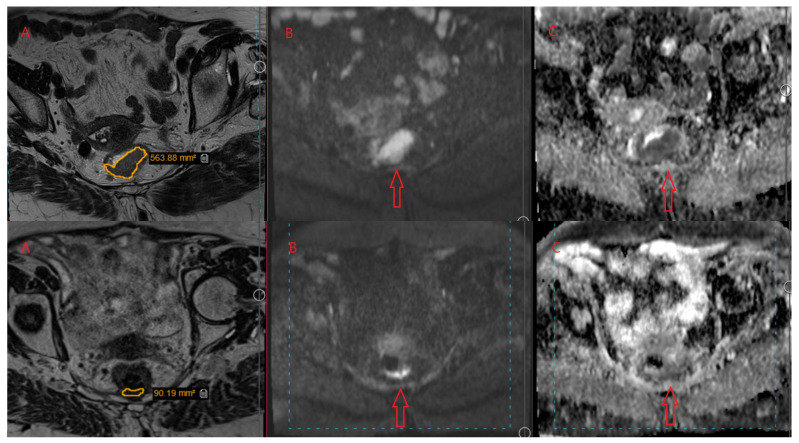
Represent MRI of rectal cancer before (upper pictures) and after treatment (lower pictures) in the same transverse section: Region of Interest (ROI) was sketched in yellow line on T2W images, marked tumor with red arrow in DWI images and ADC map images. (A) = T2W image; (B) = DWI image; (C) = ADC map image.
